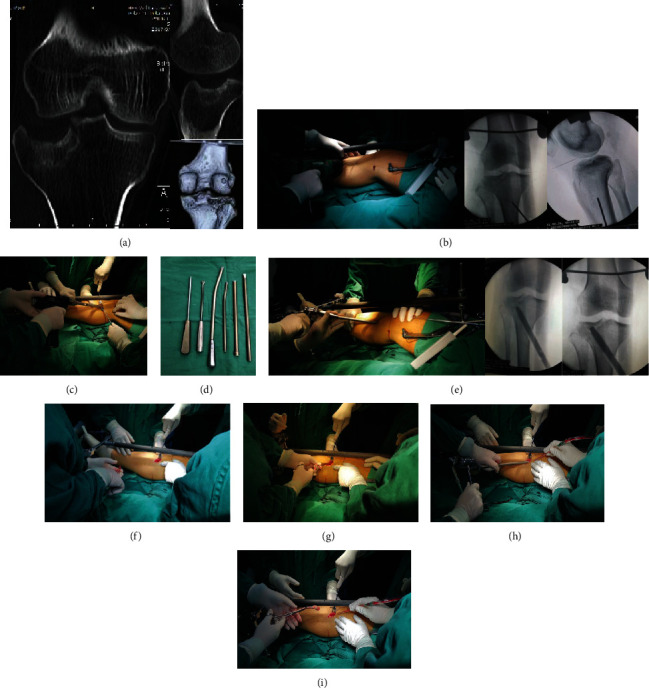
Steps of the reduction of the depressed articular fragments via TBTM and filling of the structural bicortical autologous iliac crest bone segment. (a) Knee CT scans of a 42-year-old man with DTPF; (b) a syringe needle was used to locate the articular cavity of the knee, and a 2.5 mm Kirschner wire was used to locate the position of the depressed area; (c) a power-driven trephine was used to create a bone tunnel on the anterior proximal tibia; (d) cylindrical metal tamps of different shapes and sizes; (e) retrograde reduction of the depressed articular fragments; (f) after reduction, cancellous bone was filled beneath the depressed articular fragments; (g)–(i) the structural bicortical autologous iliac crest bone segment was placed and compressed with a metal bone tamper. Then, reconstruction of the tibial cortical bone window was performed.
